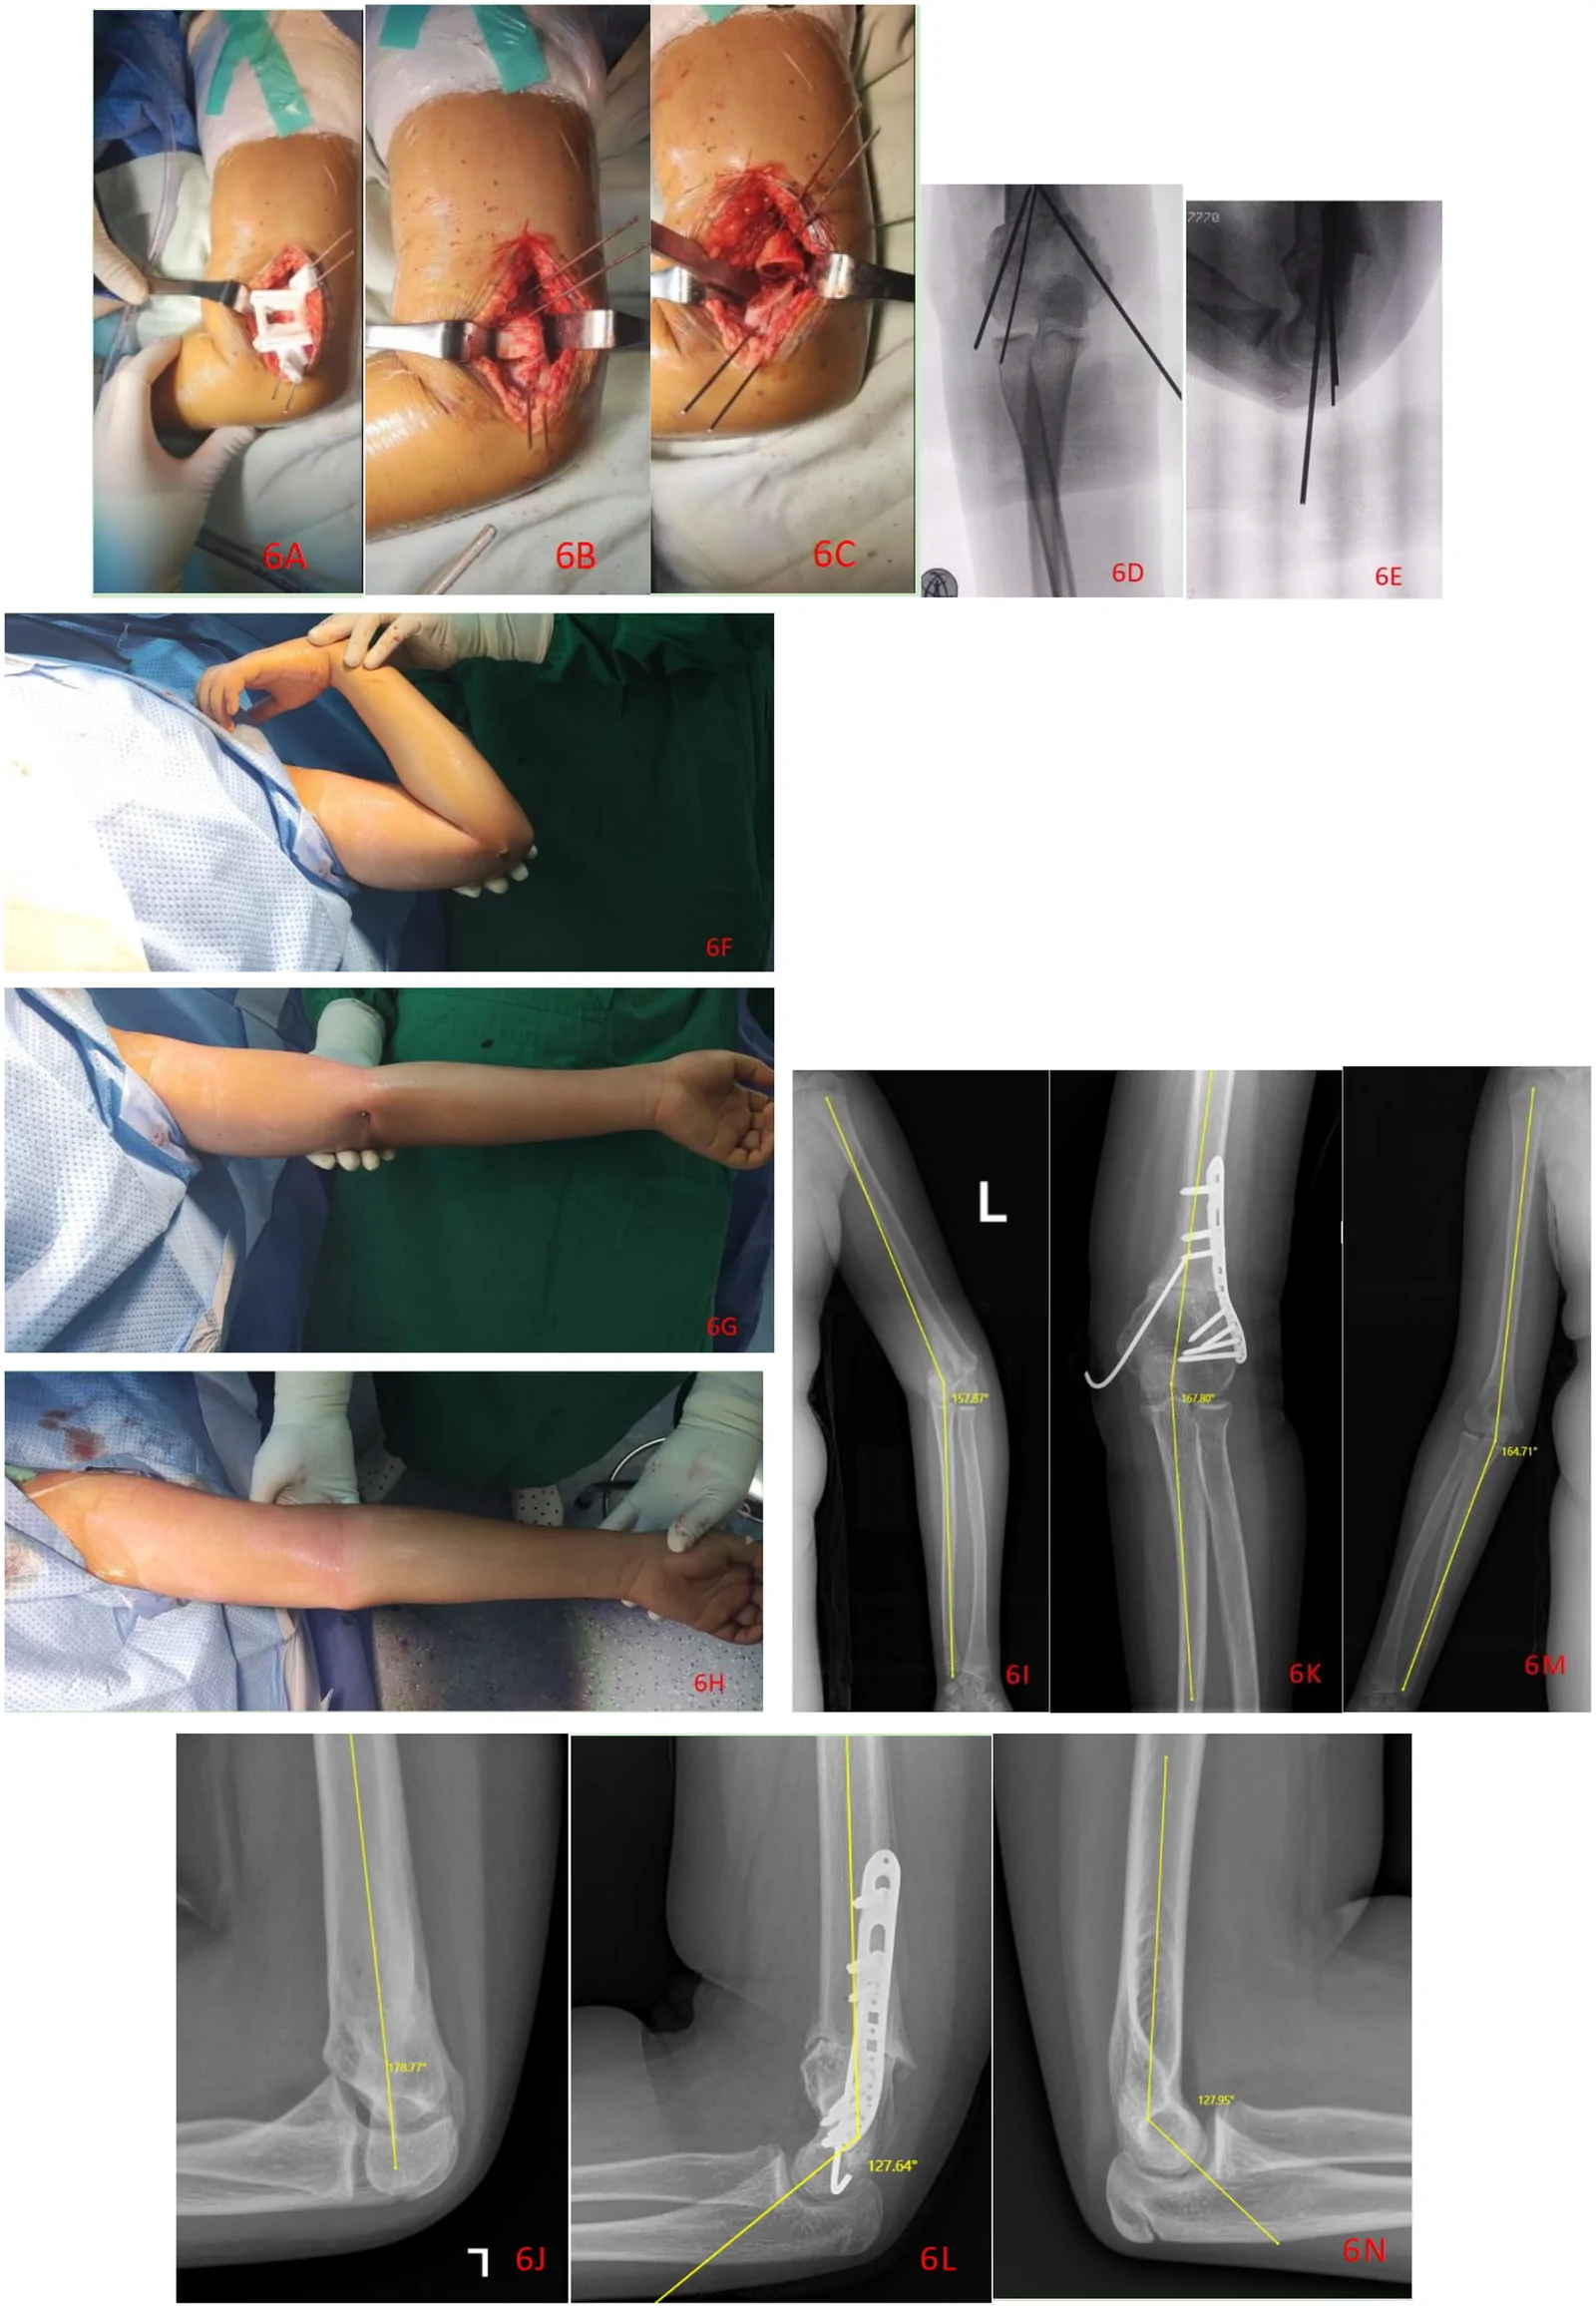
Intraoperative use of custom-made templates and postoperative radiographs. (A) Osteotomy template positioned and fixed with K-wires. (B) Osteotomy performed through slits. (C) Wedge-shaped bone removed. (D,E) Temporary fixation with reduction template and additional K-wires. (F–H) Normal passive range of motion immediately postoperatively. (I–N) Preoperative and postoperative AP and lateral x-rays showing accurate correction.
